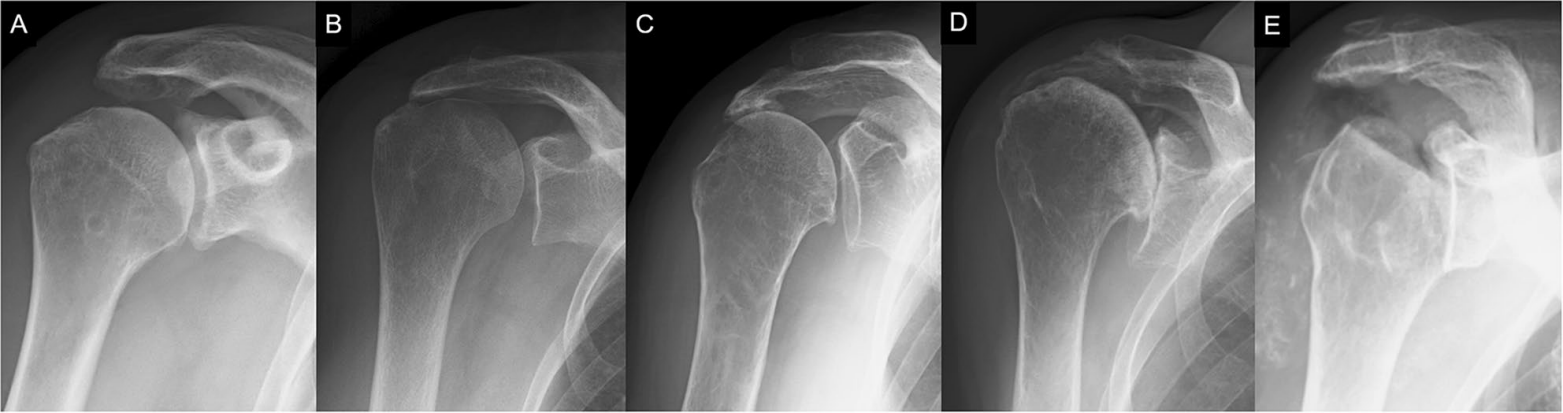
Radiographic images showing different grades of the Hamada classification of massive rotator cuff tears. (A) Grade 1 is characterized by a maintained acromiohumeral interval. (B) Grade 2 shows narrowing of the acromiohumeral interval. (C) Grade 3 shows subacromial acetabulization in addition to grade 2 features. (D) Grade 4 shows narrowing of the glenohumeral joint in addition to grade 3 features. (E) Grade 5 indicates humeral head collapse.
